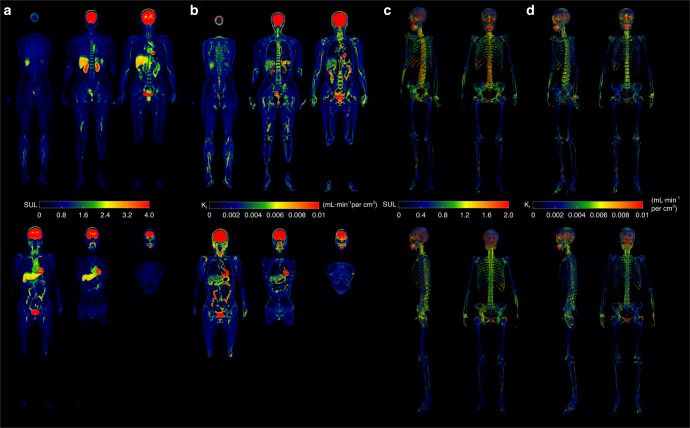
^18^F-FDG uptake profiles across the human body. a Total-body SUL map of a 51-year-old female participant. b Total-body K_i_ maps of the 51-year-old female participant. The images are displayed in the coronal view. c Skeletal SUL maps across the human body of the 51-year-old female participant. d Skeletal K_i_ maps across the human body of the 51-year-old female participant. The images are displayed in the oblique, frontal, lateral and back views
